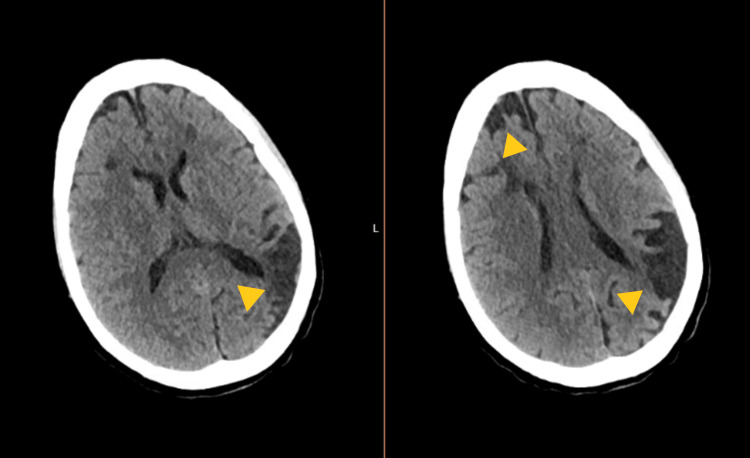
Initial CT scan head showing old infarcts and encephalomalacia
